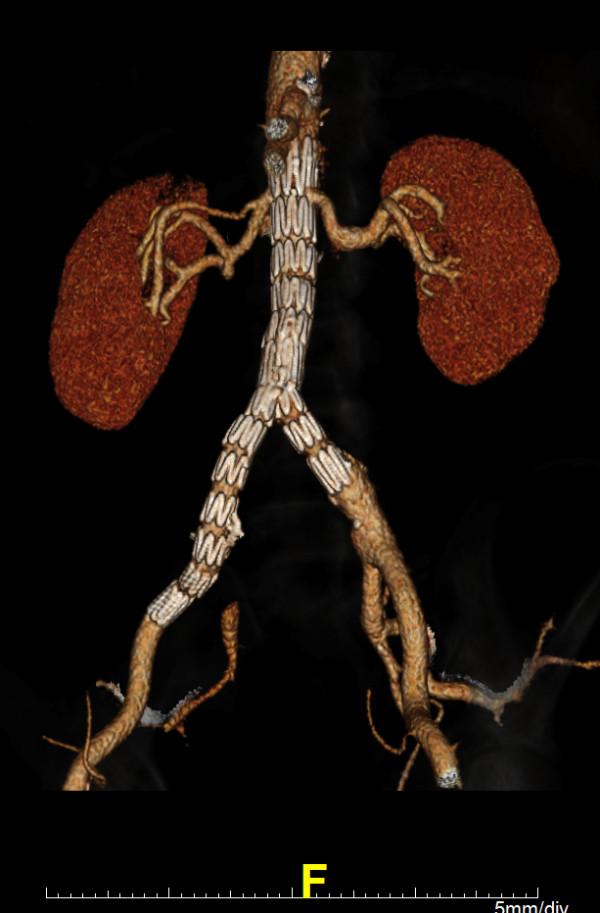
Three-dimensional volume reconstruction of a postoperative computed tomography angiogram, demonstrating an excluded right common iliac artery aneurysm with an aortoiliac stentgraft extending into the right external iliac artery and retrograde filling of the distal right internal iliac artery.
